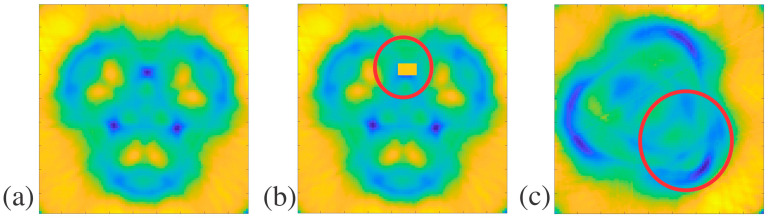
Structure A THz CT slice reconstructions in MATLAB: (a) structure A slice without defects, (b) structure A slice with an artificial defect indicated by the red circle, and (c) structure A slice with one hole filled with air indicated by the red circle.
